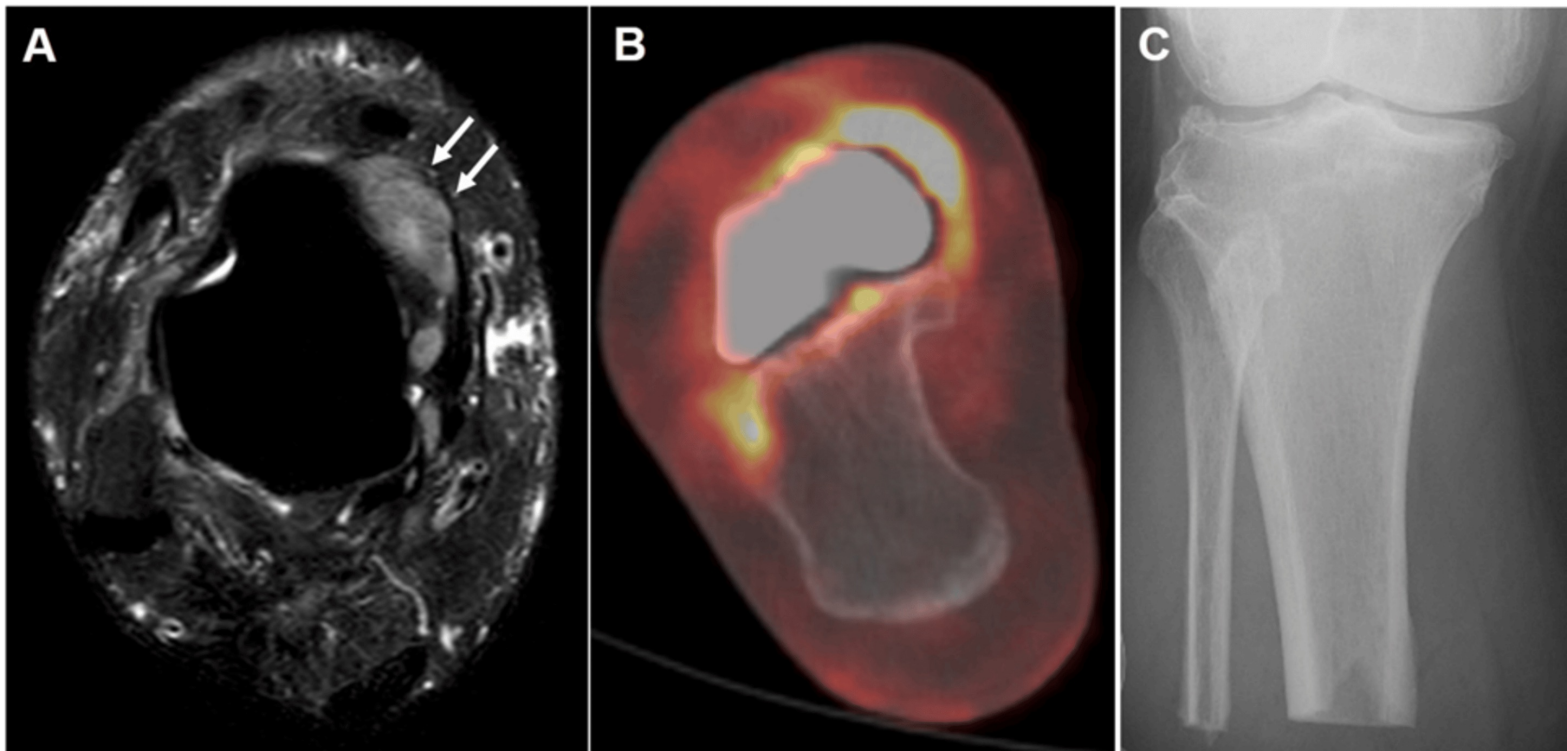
MRI and PET showed the recurrence and a below-knee amputation was performed
